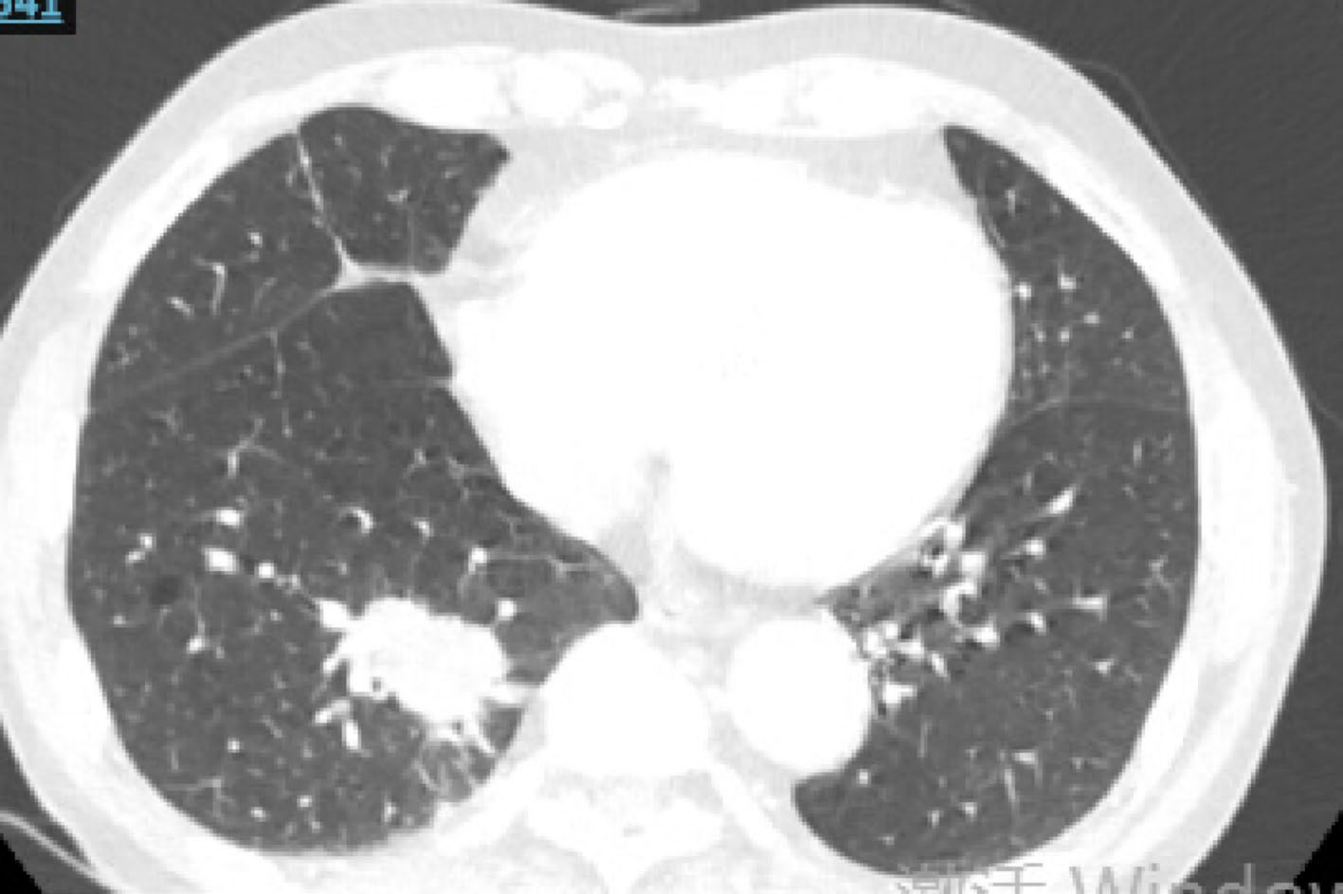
Case2 1 November 2024 CT
